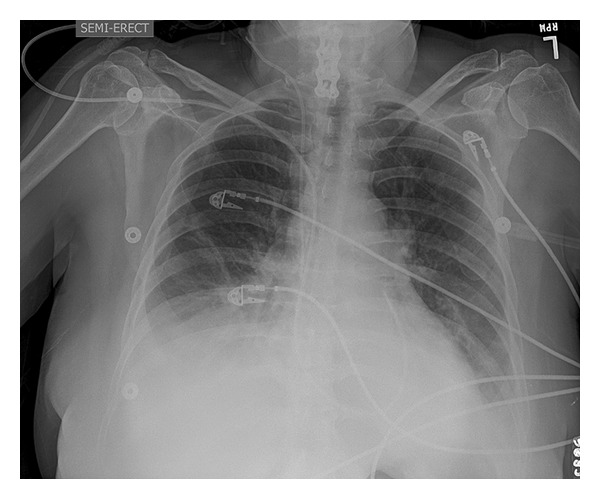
Chest plain film on presentation demonstrating likely pulmonary edema without cardiomegaly.
